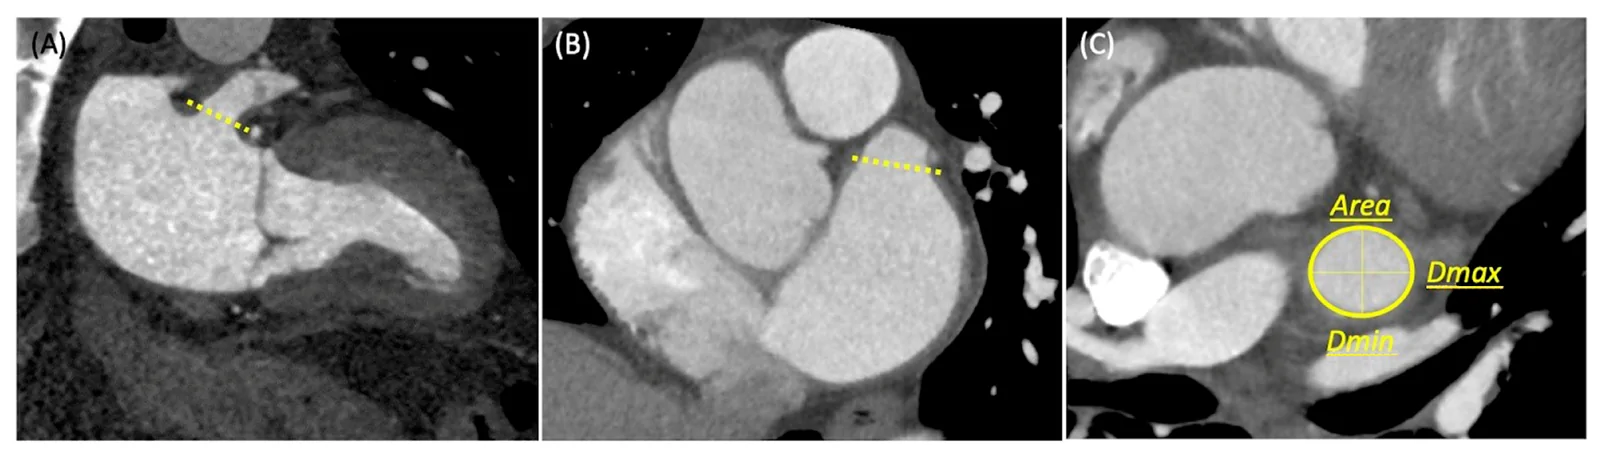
Standard CT assessment of the LAA: correct identification of the LAA ostium on two orthogonal planes of the left atrium in end-systolic images (dotted lines, panels (A,B)); measurement of ostial diameters and planimetric area for device sizing in an oblique cross-sectional plane (panel (C)).
